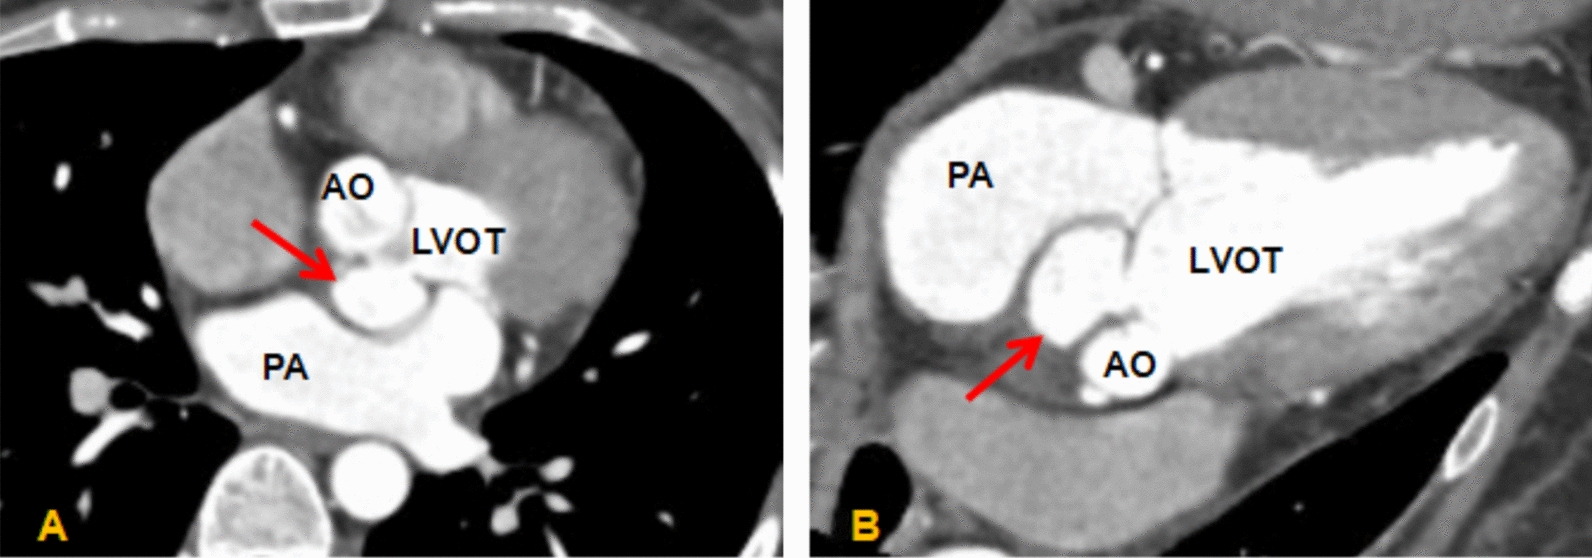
Contrast-enhanced CT. A, B Red arrows indicate the P-MAIVF cavity, confirming its communication with the left ventricular outflow tract (LVOT)
